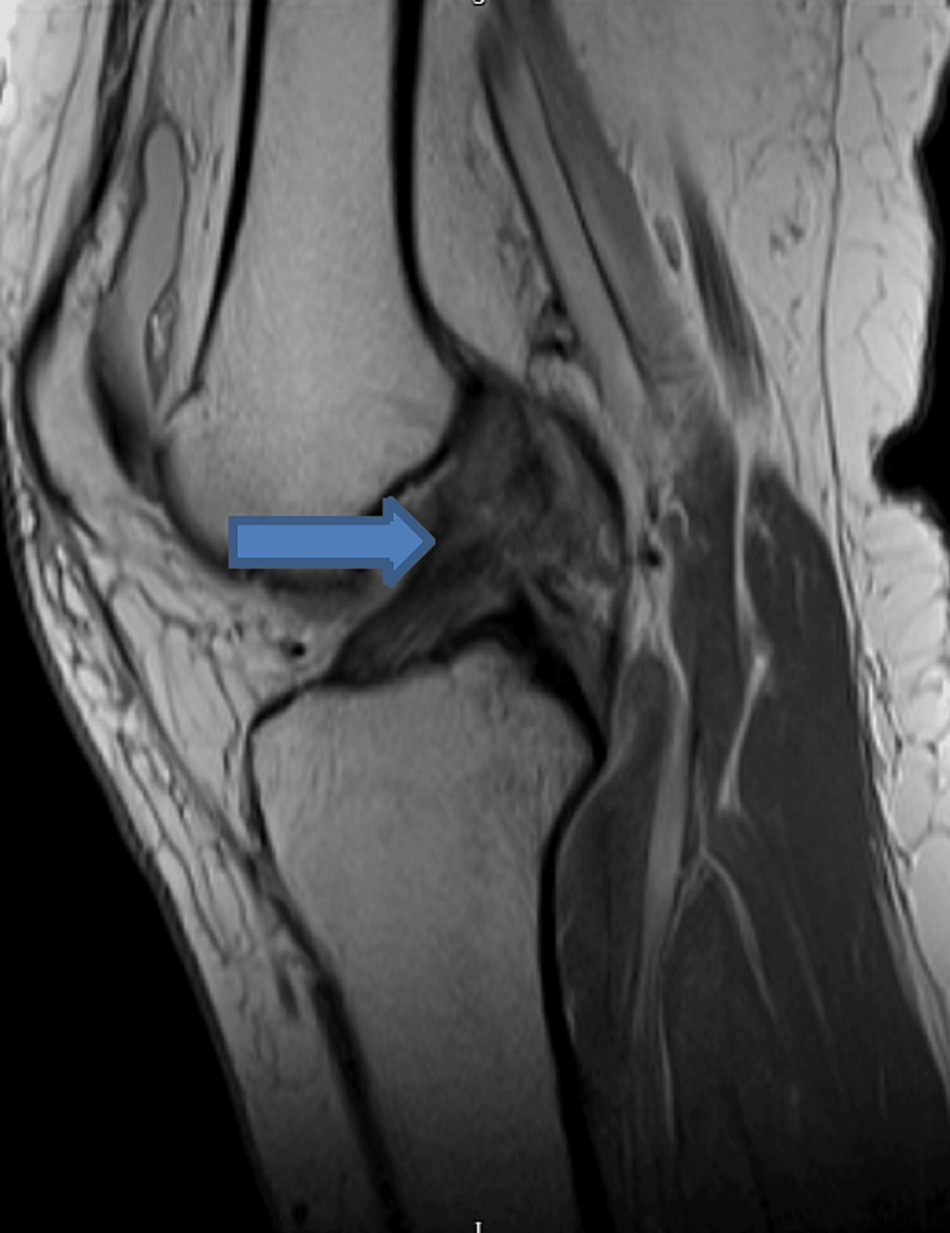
MRI scan showing ACL ligament injury MRI: magnetic resonance imaging, ACL: anterior cruciate ligament
